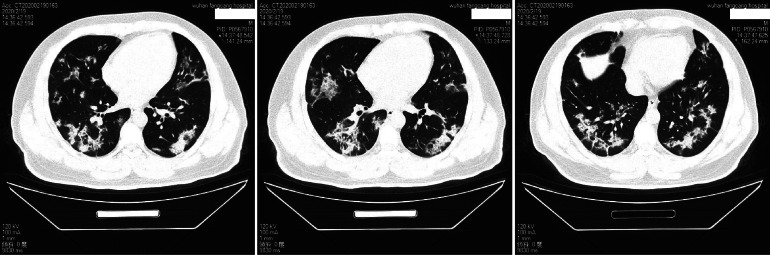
Chest CT of a patient with COVID-19. A typical CT manifestation of 40-y-old male patient, showing ground glass-like exudation in both lungs.
